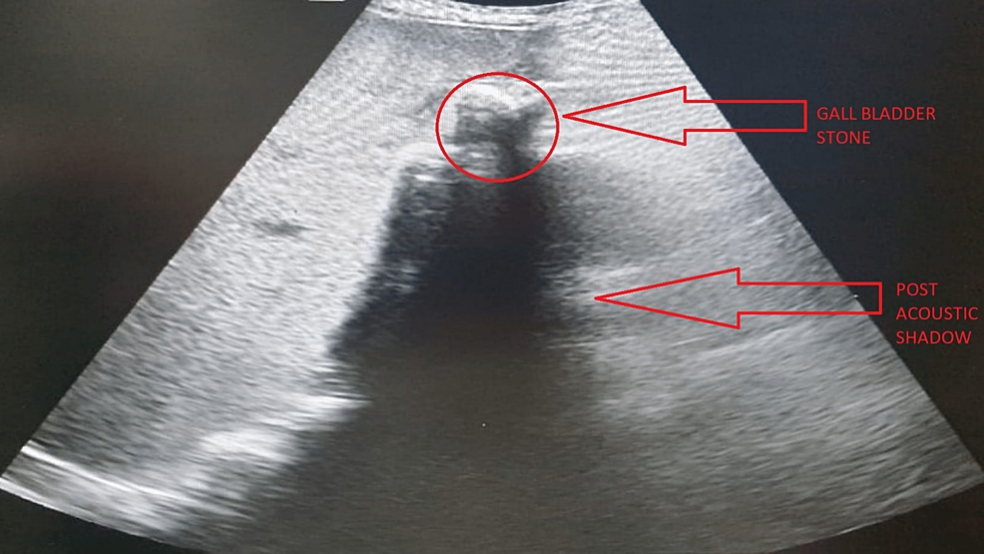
Ultrasonography image showing calculus in the gall bladder
